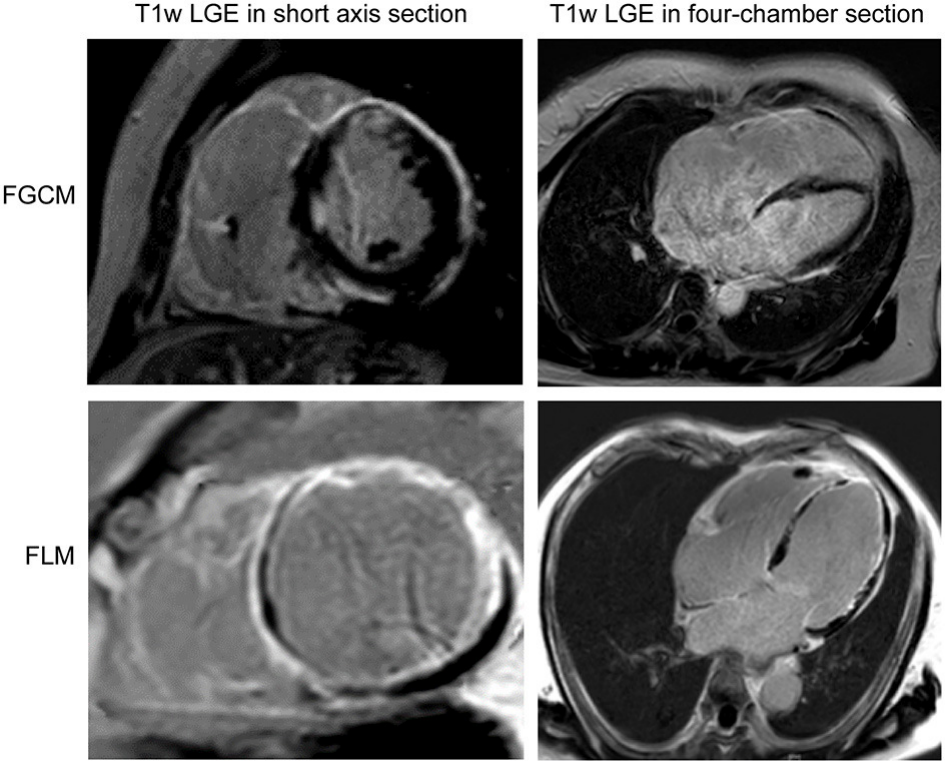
Short axis (left column) and four-chamber section (right column) cardiac magnetic resonance images of representative patients with FGCM (upper line) and FLM (lower line).
